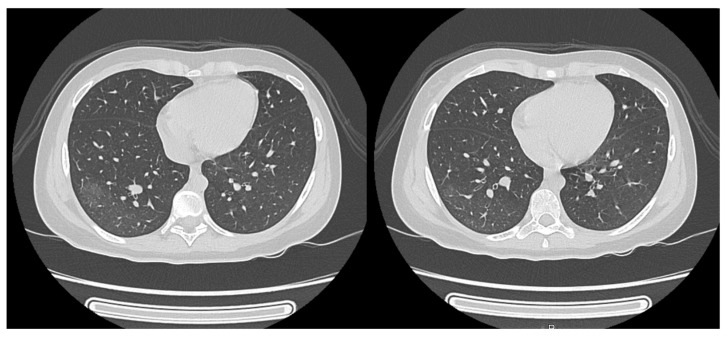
CT scan of the chest after treatment with methylprednisolone.
